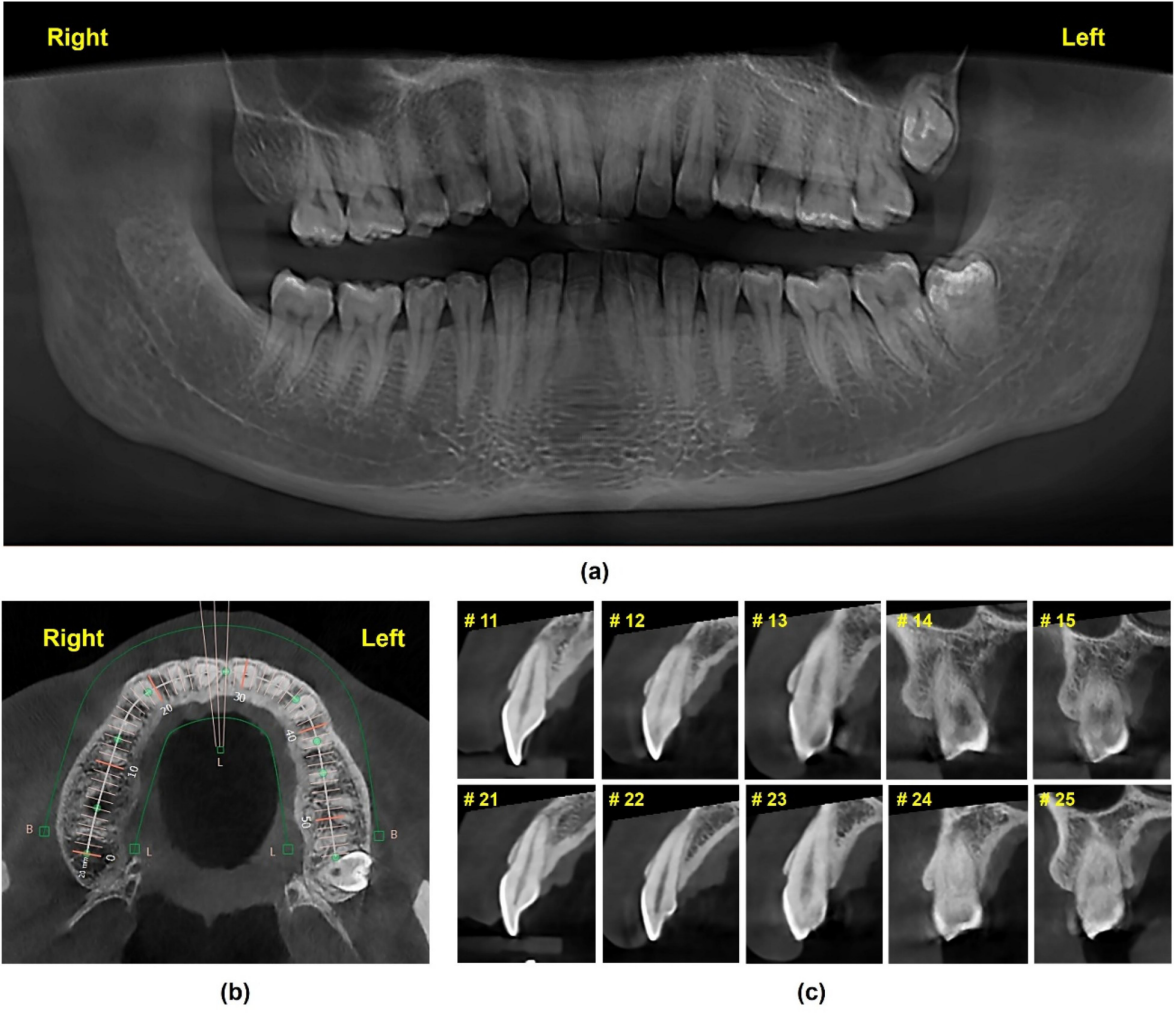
Initial radiographic data of the patient. (a) Panoramic radiograph, (b), (c) Sagittal sections of teeth #15 to #25 at Multiplanar Reformat Mode (MPR) of the Conebeam CT (CBCT) radiograph.
